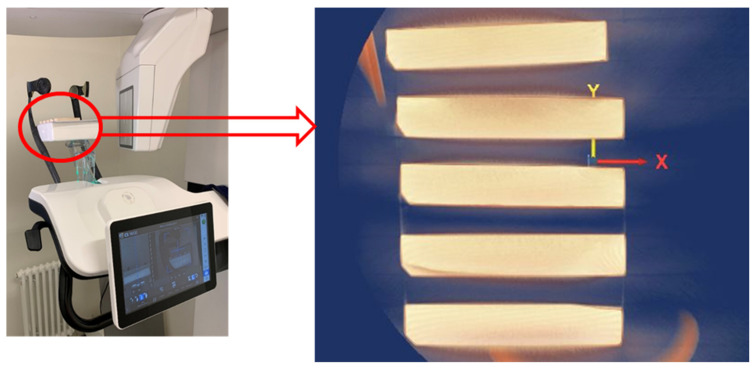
Samples prior to CT scan.
